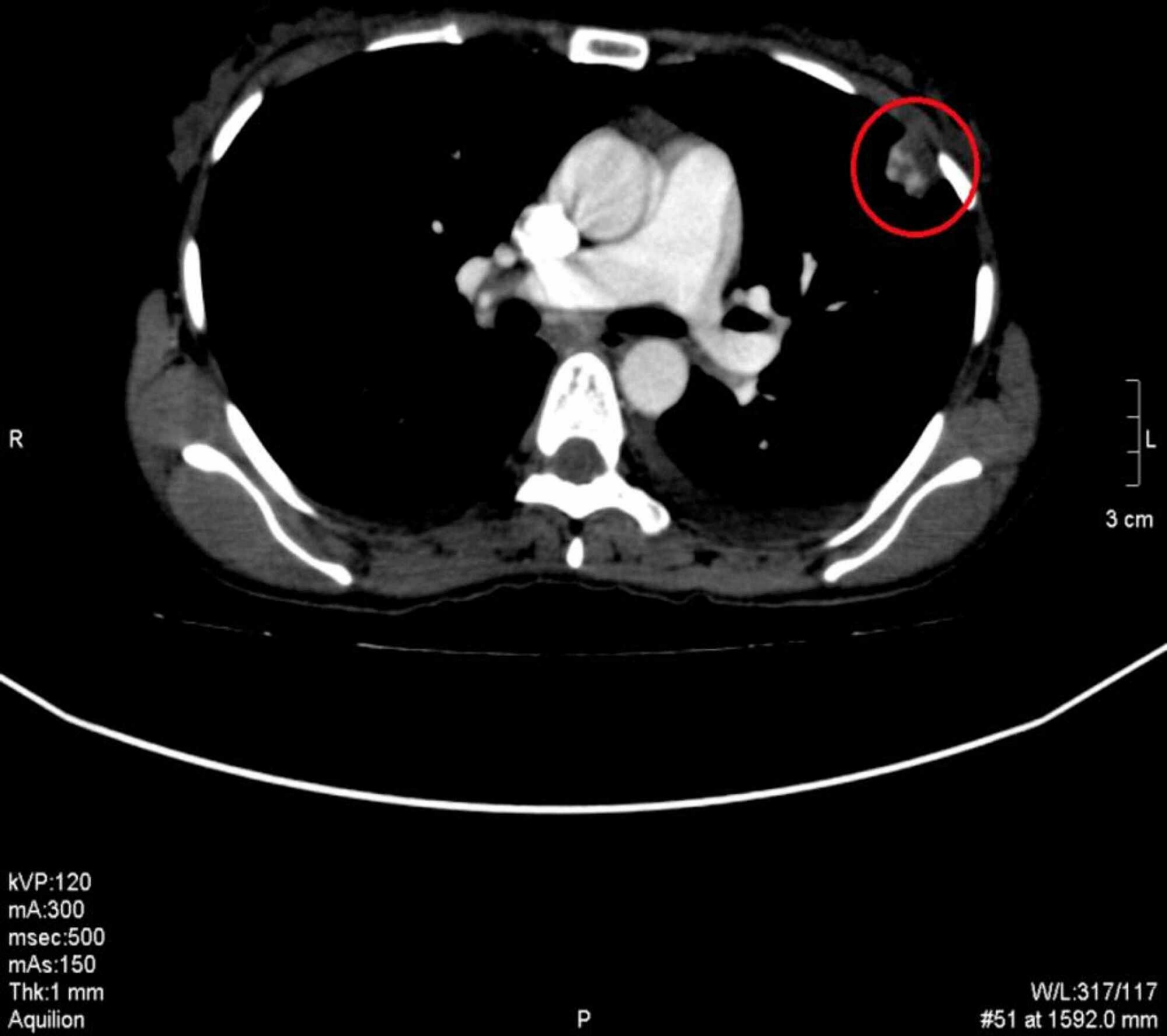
CECT chest, mediastinal window shows the vascular nature of the pulmonary nodule CECT: contrast-enhanced computed tomography
